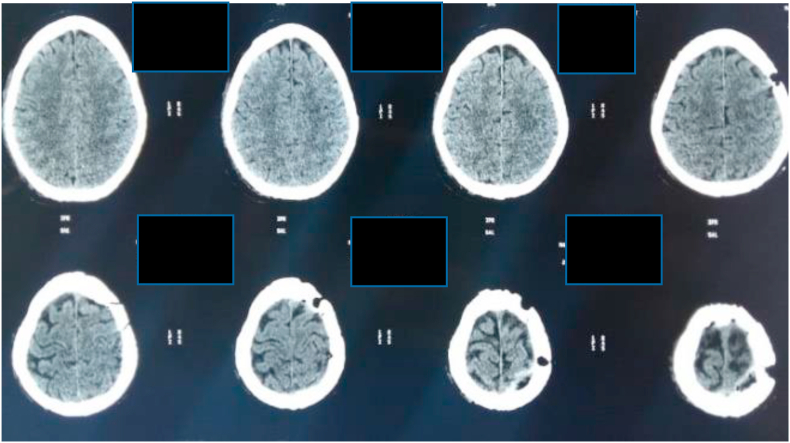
CT Scan post op showing a disappearance of the hematoma.
